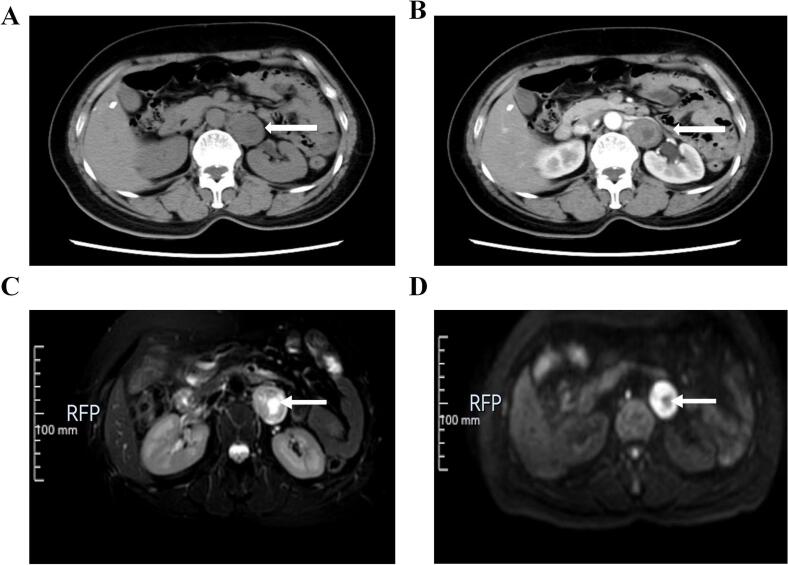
Preoperative imaging images. A. Unenhanced CT of the urinary system revealed a left retroperitoneal mass, 3.2 cm × 2.7 cm in size, with uneven density and a central hypodense lesion; B. Enhanced CT of the urinary system revealed that enhancement was heterogeneous, with no significant enhancement in the central hypodense area of the tumor; C. The results of MRI examination showed slightly high signal intensity in the center of the T2WI lesion; D. DWI showed low signal intensity in the central area of the tumor and high signal intensity in the surrounding area.
